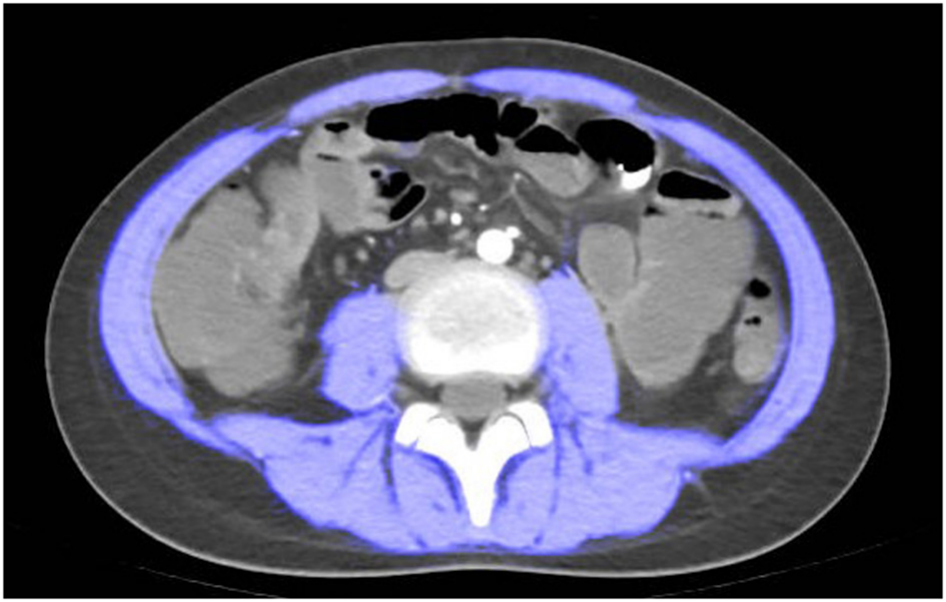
Computed tomography (CT) scan measured at spinal level L3. a patient with sarcopenia (Male, height = 1.55 m, L3SMI 28.67, L3SMI Z-score−2.423).
